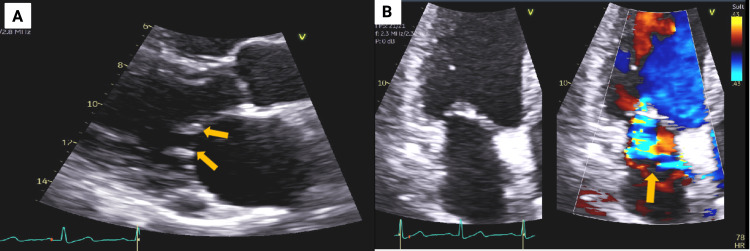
Transthoracic echocardiogram (TTE) images. The TTE was ordered after the diagnostic challenges with the TEE. Panel A demonstrates a parasternal long-axis view showing large irregular echo densities on both mitral leaflet tips, consistent with ‘kissing lesion’ (yellow arrows). Panel B shows color Doppler imaging across the mitral valve, with mild to moderate mitral regurgitation (yellow arrow).
